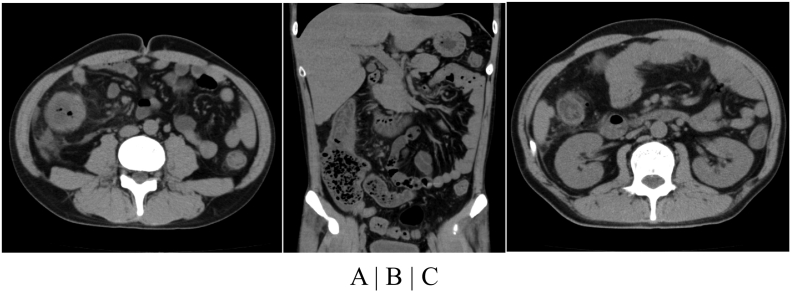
Plain CT findings. (A) A plain CT scan obtained on admission showed a thickened ascending colon wall with stenosis, a ballooning cecum, and high-density lesions in the retroperitoneum. (B) Oral intestinal distention was observed. (C) A plain CT scan obtained the day after admission showed small air bubbles besides the ascending colon without fluid collection.
